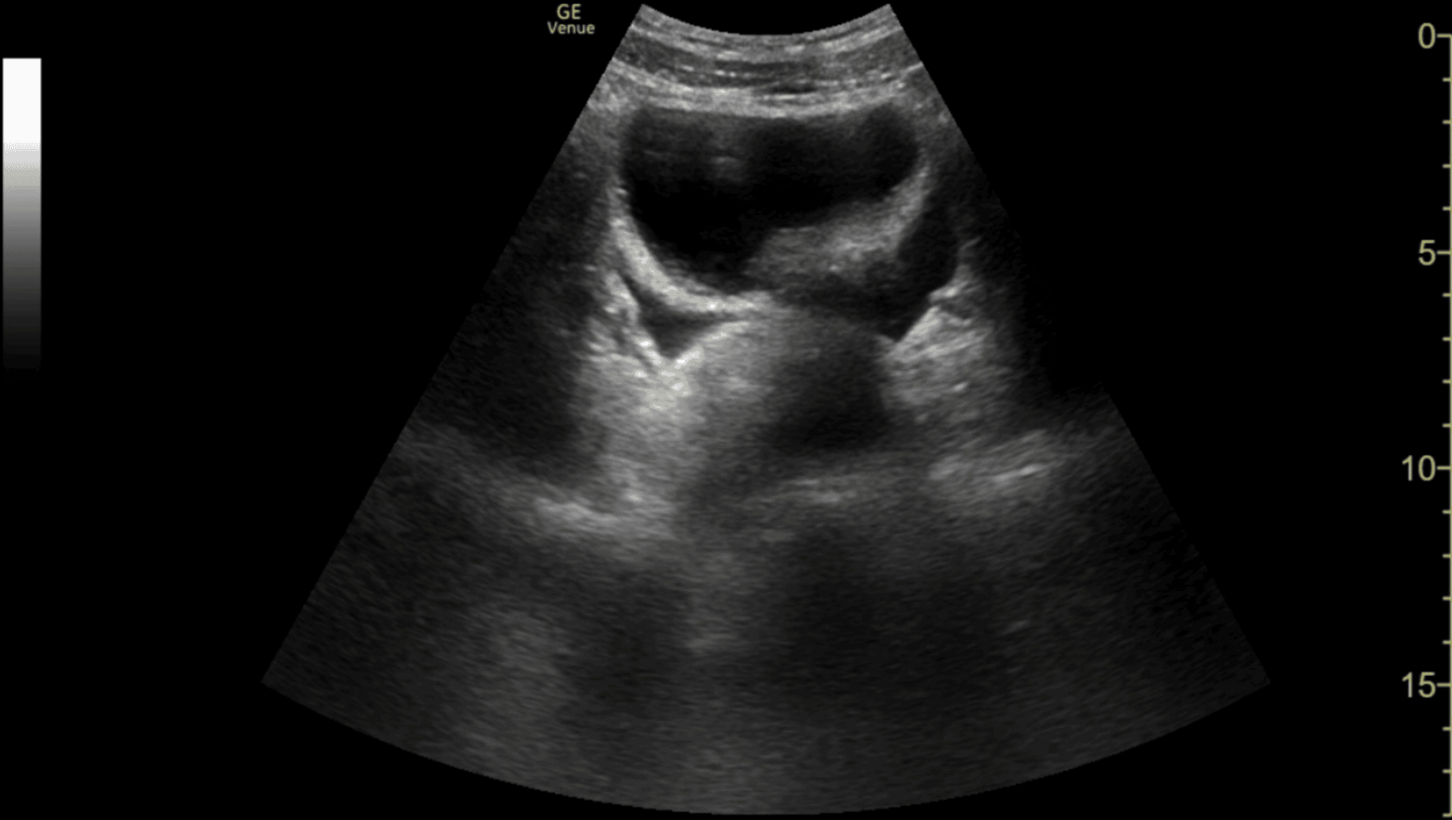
FAST scan, suprapubic transverse view, demonstrating free fluid in the pelvic space FAST: focused abdominal sonography in trauma
